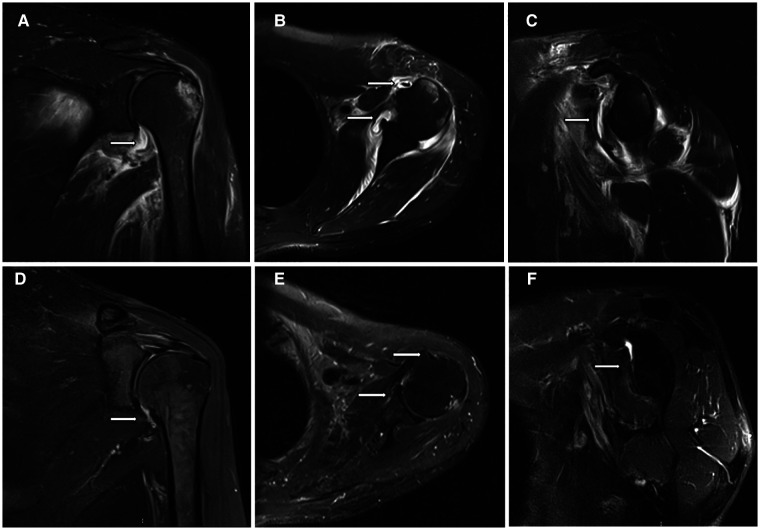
MRI findings of patients with frozen shoulder before and 1 month after arthroscopic capsular release. (A) Thickening and edema of the shoulder capsule (white arrow); (B) Edema and adhesions surrounding the long head of the biceps tendon and rotator interval (white arrow); (C) Thickening of the glenohumeral ligament (white arrows); (D) The articular capsule after release (white arrows); (E) Long head of the biceps tendon and rotator interval after release (white arrows); (F) Removal of the glenohumeral ligament (white arrow).
